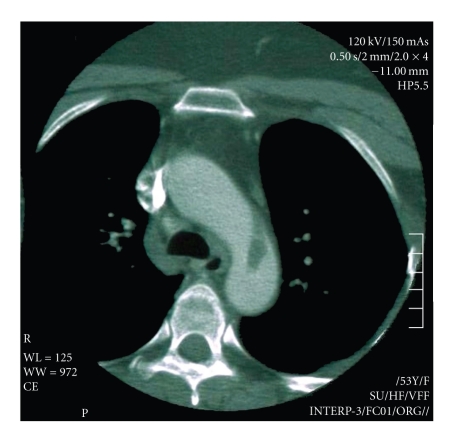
Angio-CT documenting the floating thrombus.
